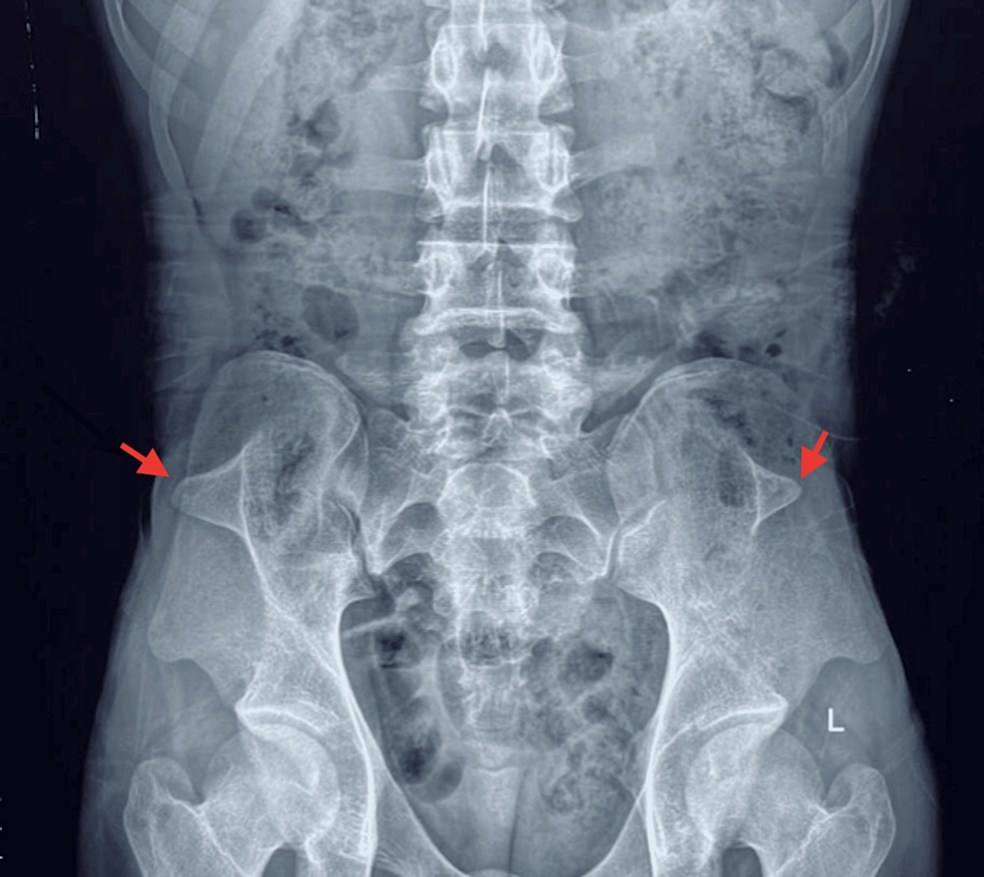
Pelvic X-ray showing bilateral iliac horns
